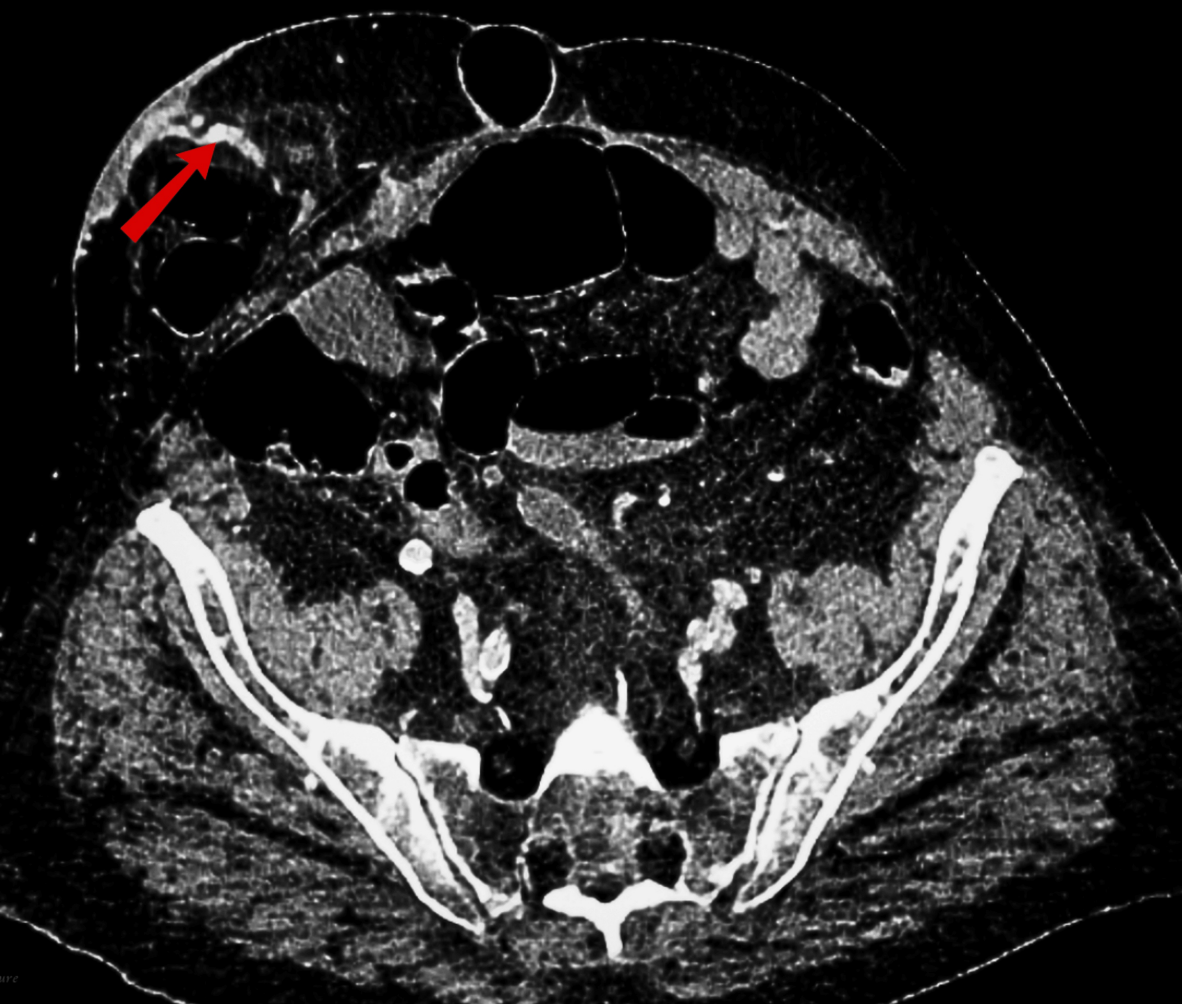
CT scan showing abnormal dilated vein arising from the conduit
